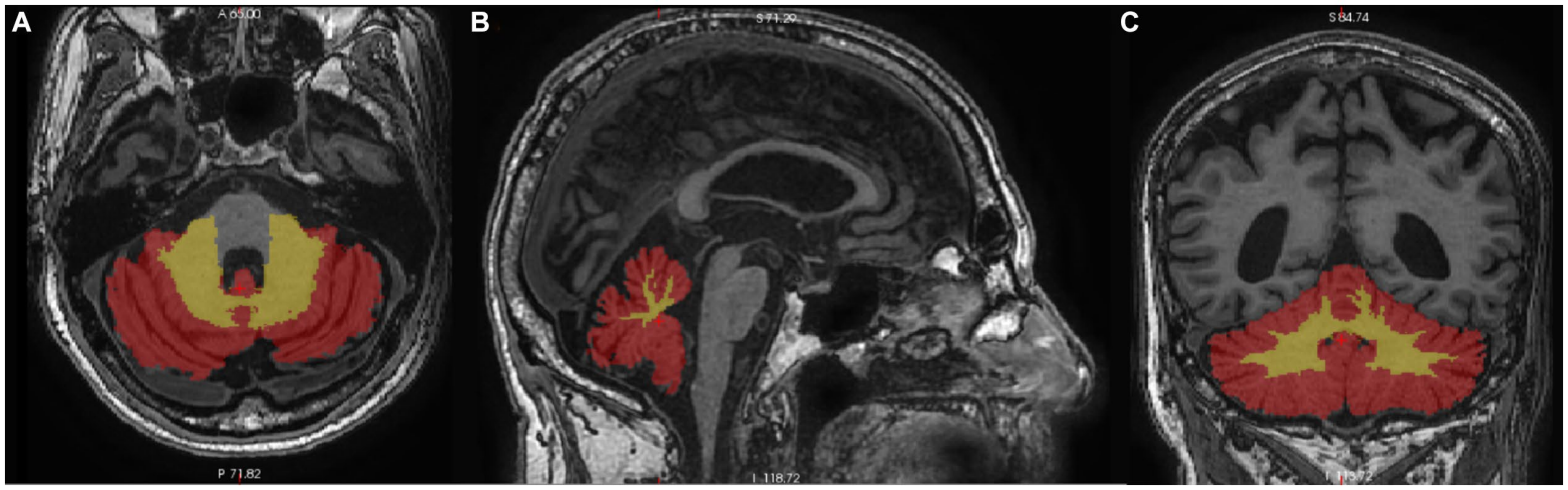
Axial (A), sagittal (B), and coronal (C) view of the cerebellum segmented on T1-weighted images. Cerebellar gray matter is colored red, and cerebellar white matter is yellow.
